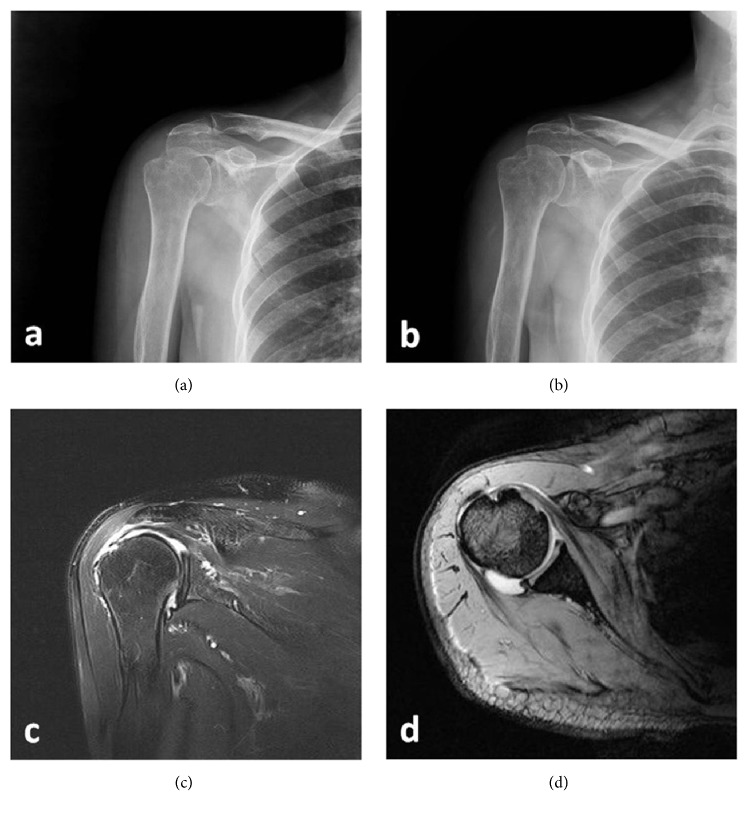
Radiographic evaluation of a 70-year-old woman with a negative culture result. (a) Preoperative standard X-ray (anterior-posterior view) showing no bony destruction of the greater tuberosity, (b) Postoperative normal proximal humerus, (c) MRI-T2-coronal view showing mild synovial thickening and joint effusion, and (d) MRI-T2-axial view showing joint fluid collection and mild synovial thickening.
